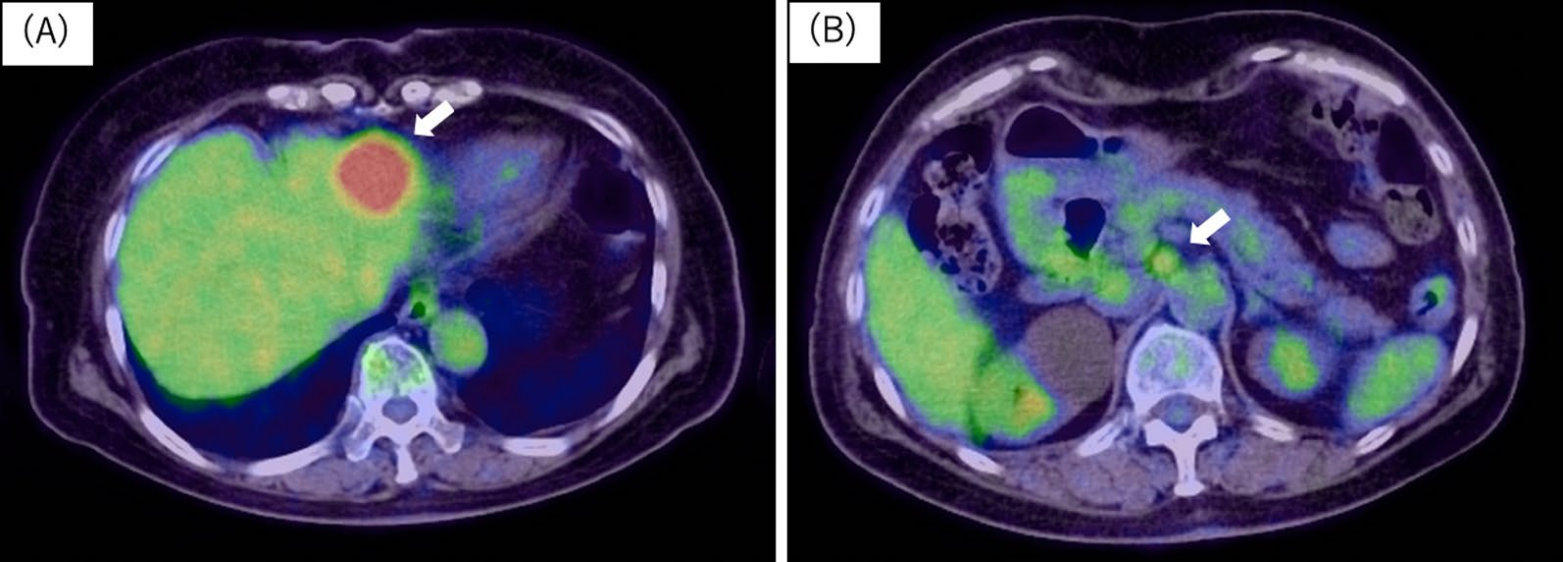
Preoperative PET–CT findings. A, B PET–CT showed the liver tumor and the retroperitoneal tumor as intense FDG uptake lesions
